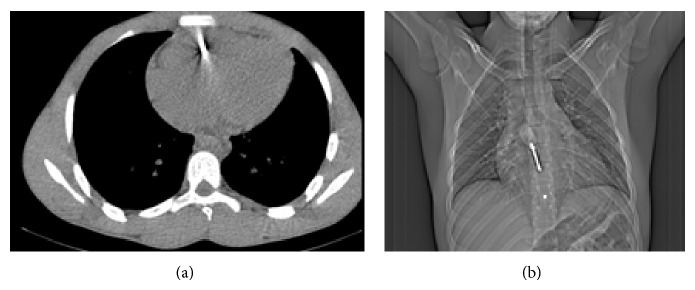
CT scan images of screwdriver inside the heart.
